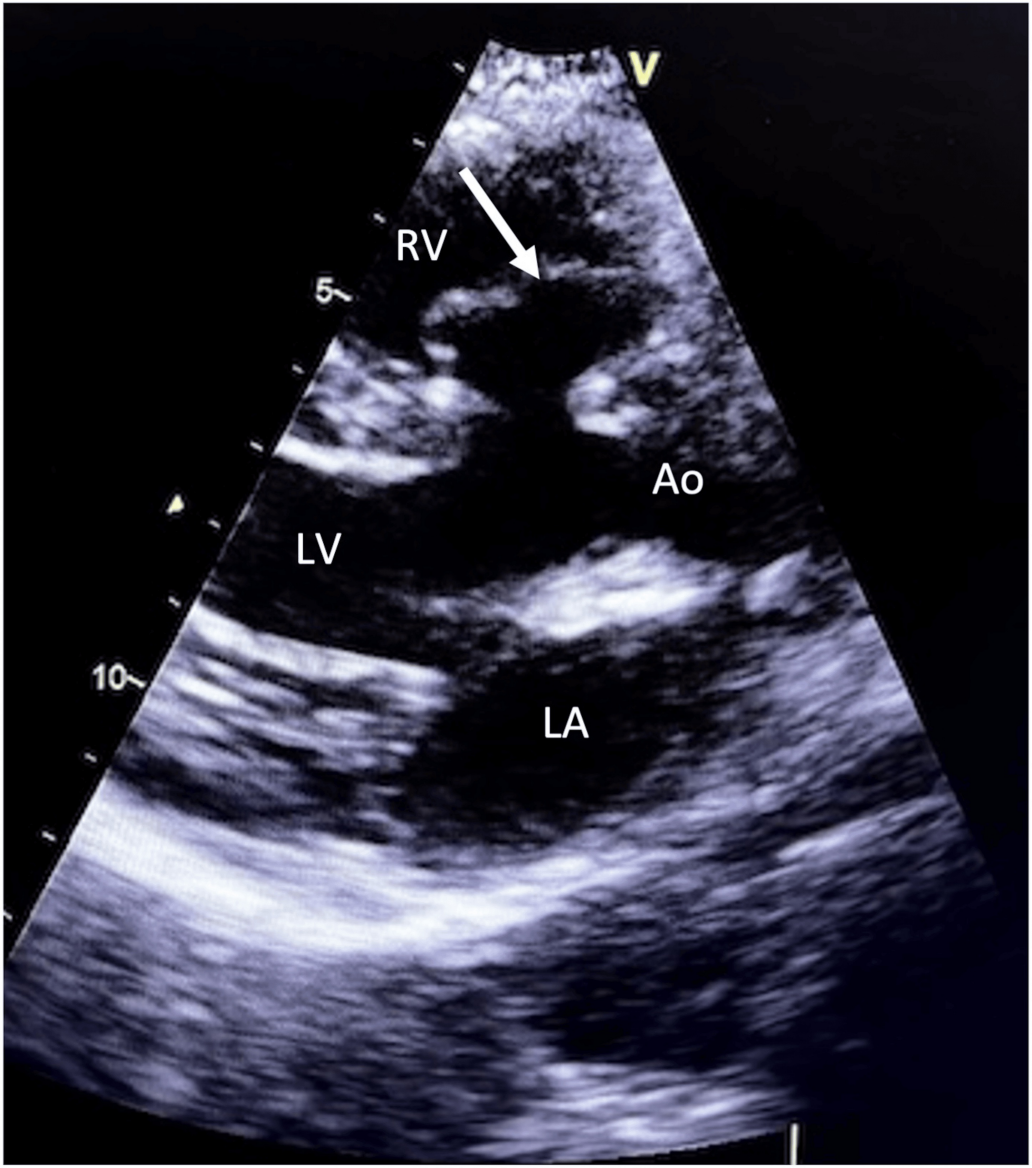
Transthoracic parasternal long-axis view echocardiography showing the membranous ventricular septal aneurysm (arrow). Ao: aorta; LA: left atrium; LV: left ventricle; RV: right ventricle.
